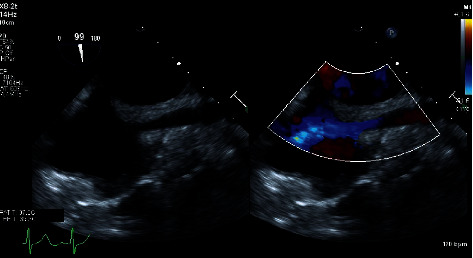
The image in the left panel shows a two-dimensional presurgical midesophageal bicaval view obtained via transesophageal echocardiography (TEE) which demonstrates mild narrowing of the SVC at the SVC-right atrial junction. The image in the right panel is the same with the addition of color flow Doppler demonstrating no flow acceleration from the superior vena cava into the right atrium.
